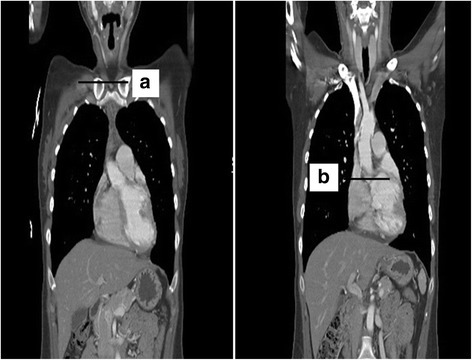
Coronal planes of cardiac computed tomography showing the level of the upper border of the sternal clavicle (a) and the level of the junction between the superior vena cava and right atrium (b)
